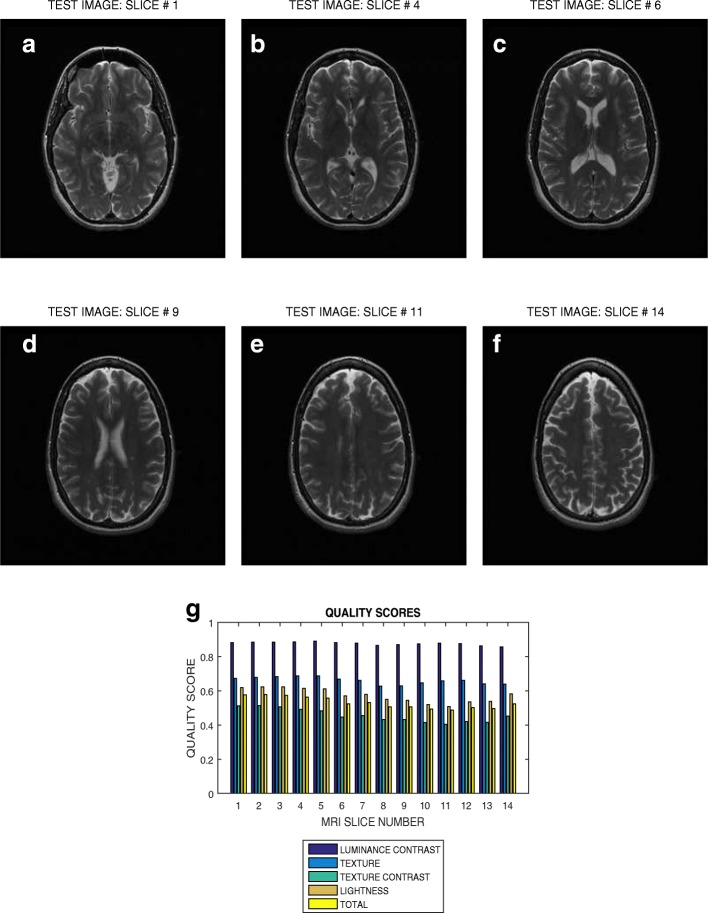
Six slices with indices (a) 1, (b) 4, (c) 6, (d) 9, (e) 11 and (f) 14 in a T2 weighted MRI volume data from NeuroRx Research Inc, (g) luminance contrast, texture, texture contrast, lightness and total quality scores of 14 successive slices in the MRI volume data
